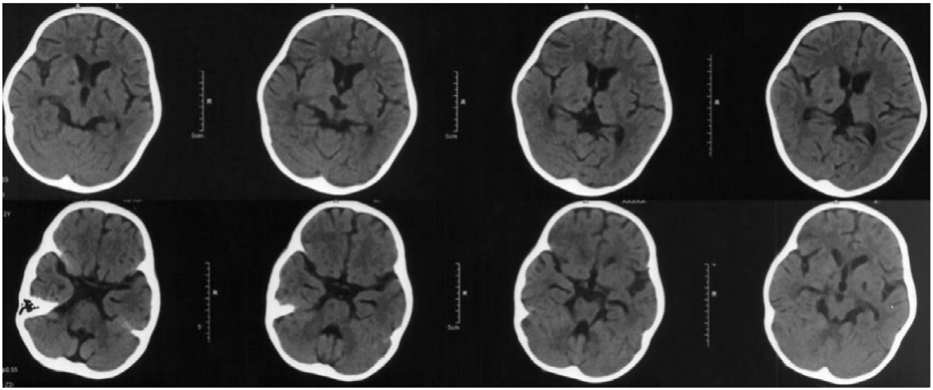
Brain CT taken at 2 years and 4 months old, and 2 weeks after disease onset: multiple spotty and patchy low-density lesions in the bilateral basal ganglia and thalamus.
